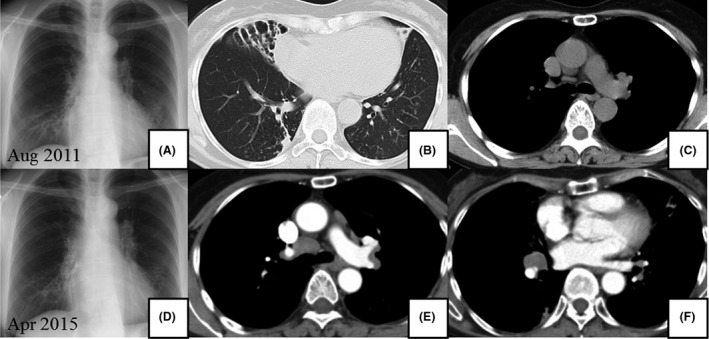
(A–C) Chest X‐ray and CT on the first visit revealed bronchiectasis and centrilobular small nodules. (D–F) Chest X‐ray and CT in April 2015 revealed bilateral hilar and mediastinal lymphadenopathy, particularly in the area of the middle lobe.
